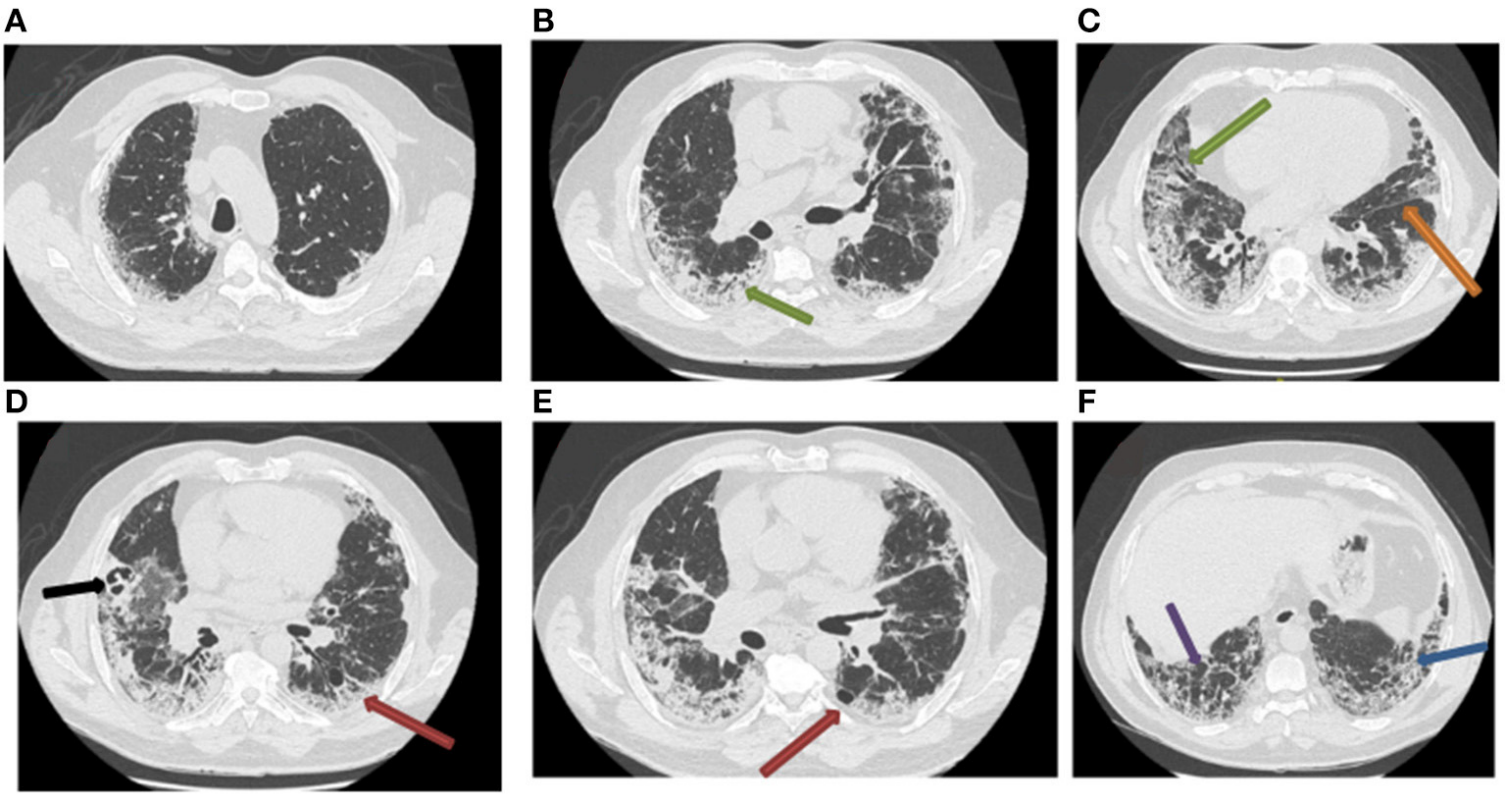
Follow-up chest CT scan of post-COVID-19 pneumonia (second chest CT scan). The chest CT scan performed 4 weeks after the management of severe COVID-19 pneumonia reveals the appearance of bronchiectasis (A–C; green arrow), subpleural reticulations (F; blue arrow), lobular distortions (F; violet arrow), fissure deformity (C; orange arrow), paracicatricial emphysema (D,E; red arrow), and cavitation in the right middle lobe (D; black arrow).
